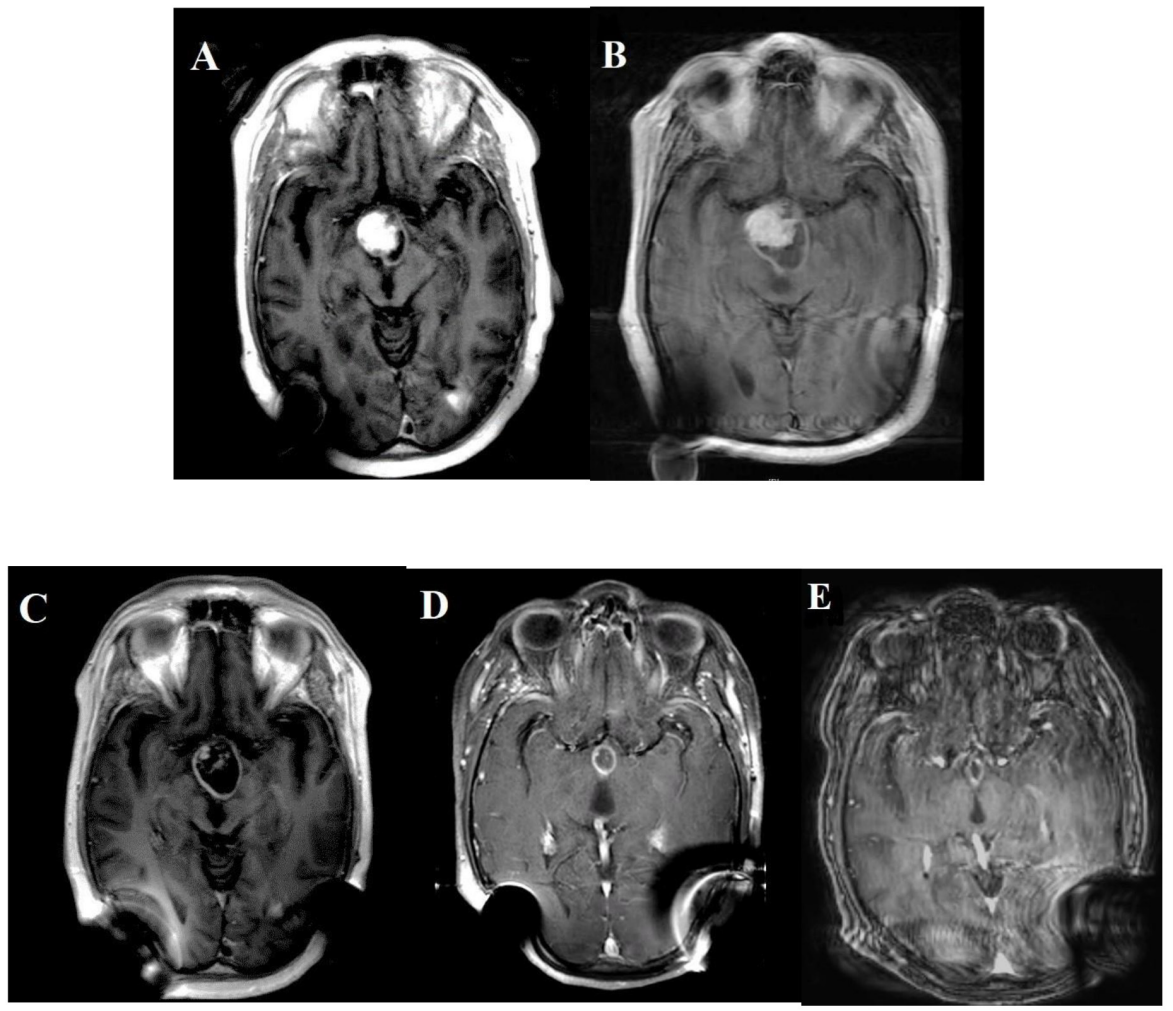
MRI brain before BRAF inhibitor was introduced (both A & B) B/L posterior distortions are the result of B/L shunts for hydrocephalus (left) and (right) respectively. MRI on the right (B) showed an increase in the size of the residual suprasellar craniopharyngioma (48 x 53 x 29 mm) predominantly due to an increase in the size of the cystic component. After BRAF inhibitor was introduced, MRI brain was done at two-month intervals. MRI in the bottom left (C) shows partial response with a significant reduction in the solid tumor. MRI in the bottom middle (D) was a year after treatment. It shows the patient's solid internal nodular enhancement, and his cyst completely resolved as compared to the original MRI (A) in the top left from February 2016. Starting May 2017, the MRIs were performed every six months. The right bottom MRI (E) continued shrinkage of the patient's suprasellar cystic lesion. It was two years after being on single-agent Dabrafenib.
